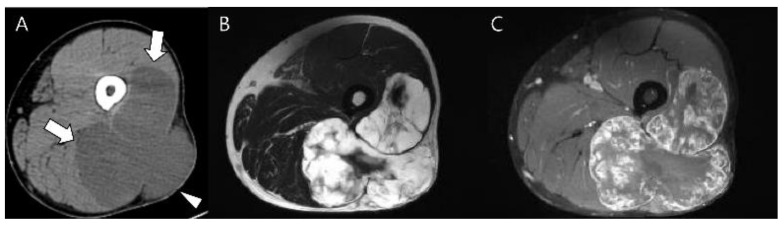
Extraskeletal myxoid chondrosarcoma of the right thigh in a 46-year-old man. (A) Axial CT scan reveals a lobulated, low-density soft tissue mass (arrows) without chondral-type mineralization between the vastus lateralis and biceps femoris muscles extending to the subcutaneous fat layer (arrowhead). (B,C) Axial T2-weighted and T1-weighted enhanced images show a soft tissue mass with high signal intensity and peripheral rim and septal enhancement.
